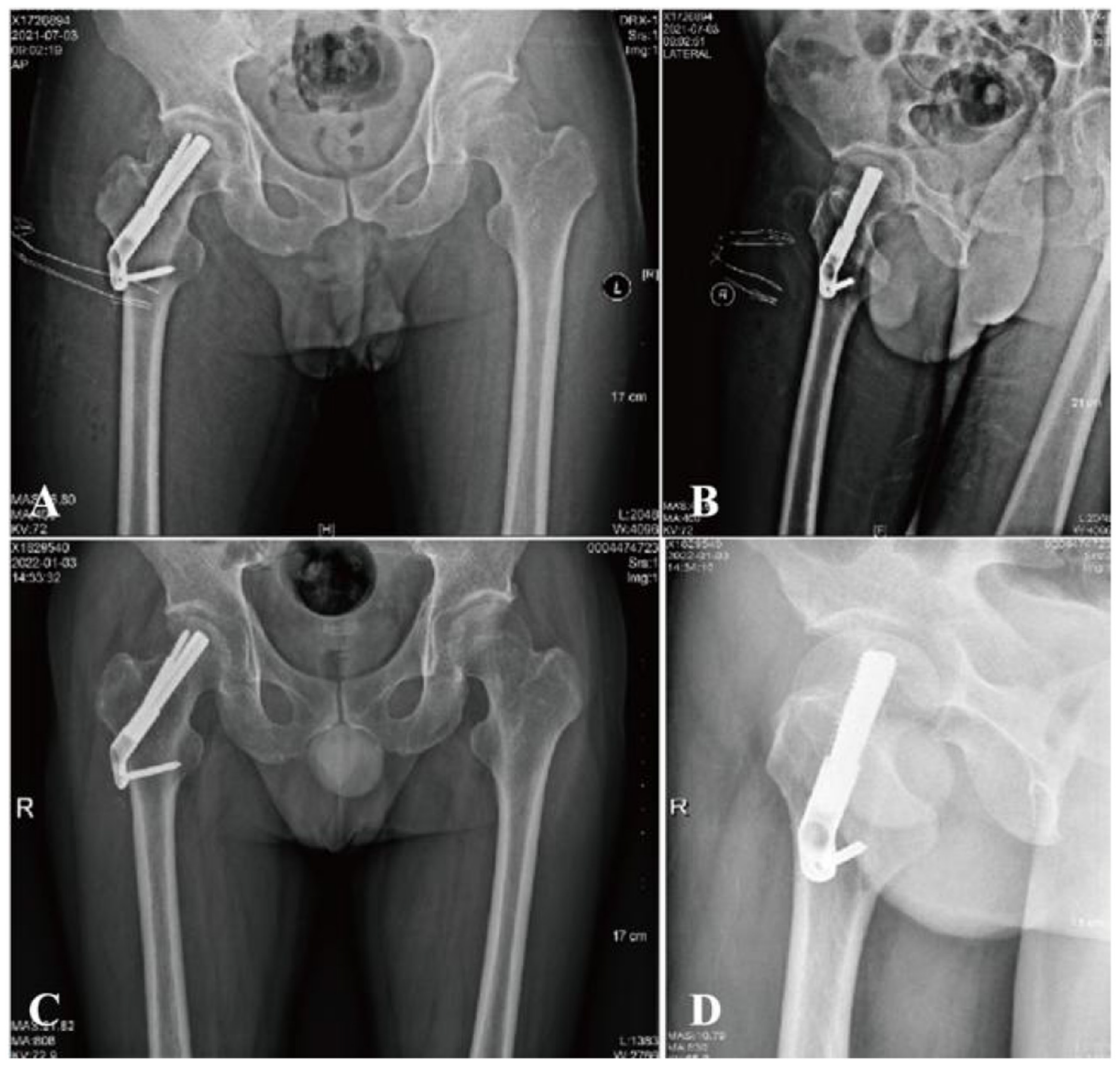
A 36-year-old male with femoral neck fracture. (A, B) Postoperative radiographs show good reduction (Garden Index I). (C, D) At 6 months, films confirm fracture healing with no shortening or avascular necrosis.
